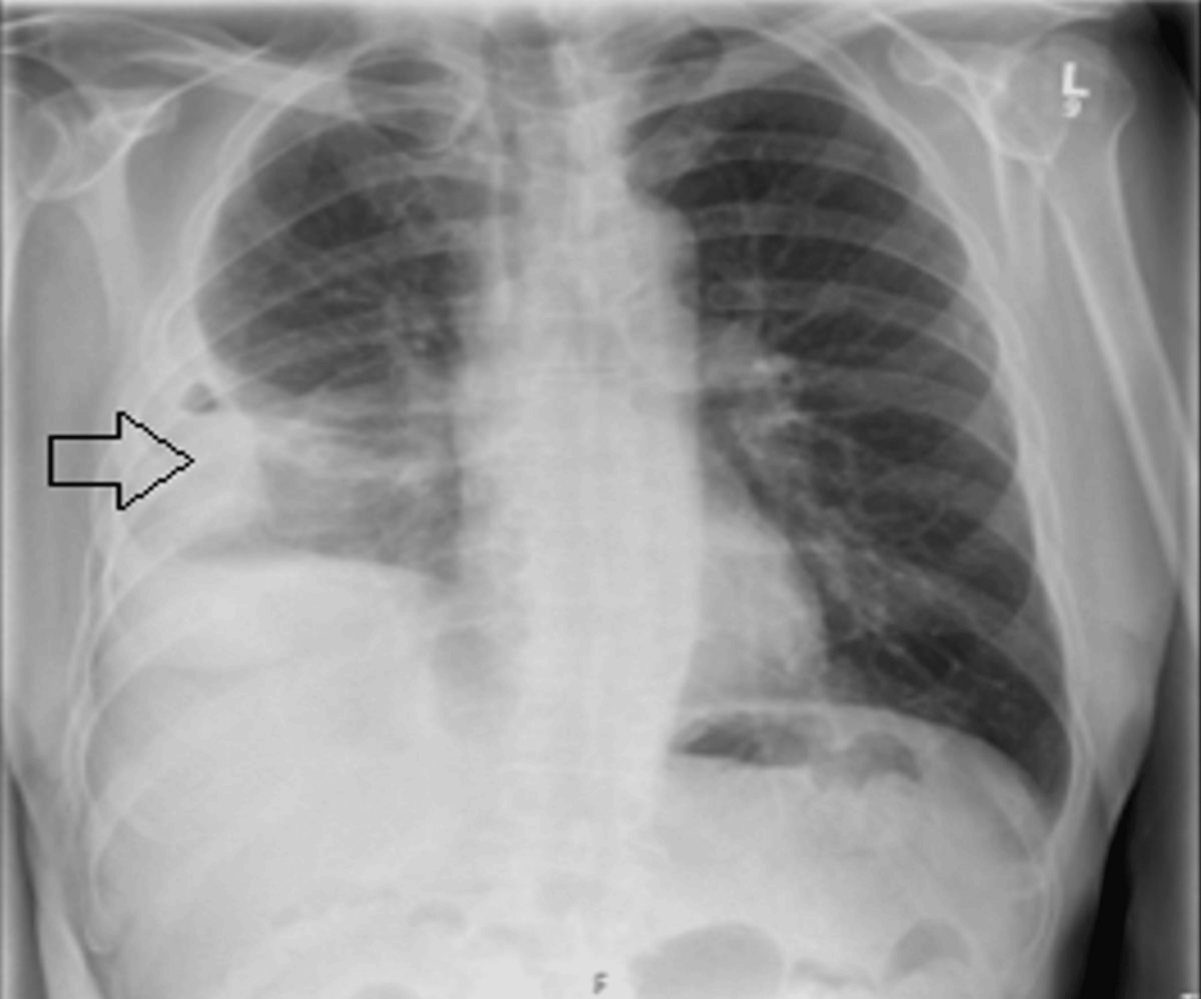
The chest X-ray revealed reaccumulation of small right-sided pleural effusion, likely secondary to trapped lung.
